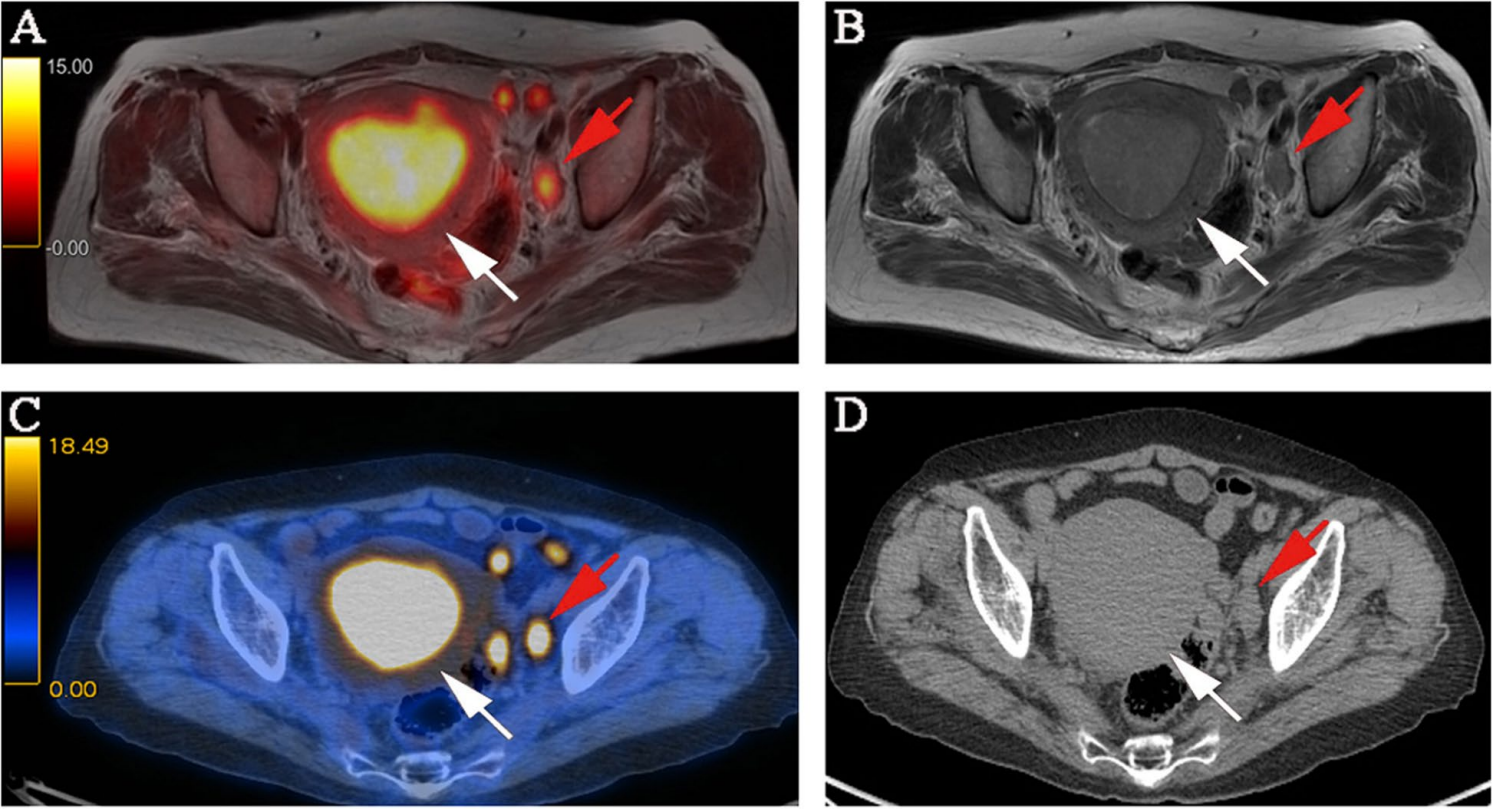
FIGO stage IIIC_1_, pathologically confirmed moderately differentiated adenocarcinoma in a 48-year-old female patient. A PET/MR images. B MR T2-weighted images. C PET/CT images. D CT images. Both PET/MRI and PET/CT show swelling of the left pelvic lymph node and high FDG uptake. The PET/MRI and PET/CT staging were consistent with the pathological stage, namely FIGO stage IIIC_1_. The white arrow indicates endometrial carcinoma, and the red arrow indicates metastatic lymph node. FIGO, International Federation of Gynecology and Obstetrics; PET, positron emission tomography; MRI, magnetic resonance imaging; CT, computed tomography
